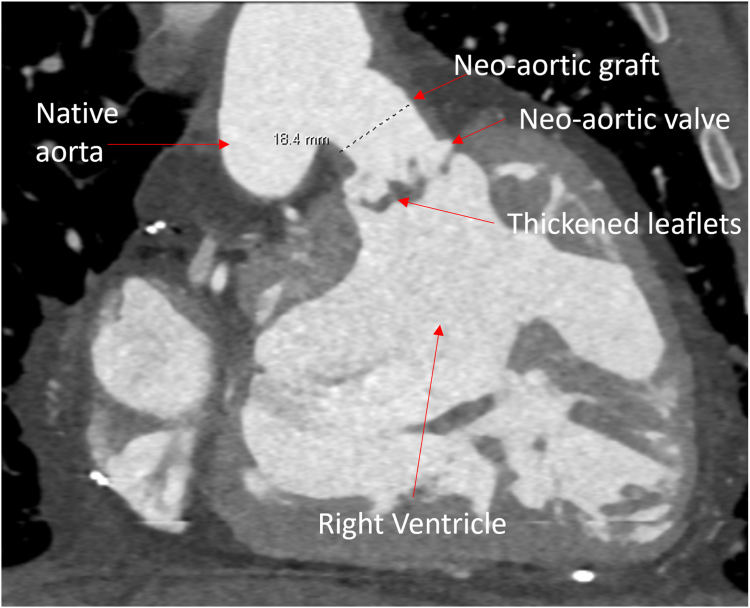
Frontal projection from cardiac computed tomography showing the native aorta and its connection with the neoaortic hemashield graft. The graft measures 18 mm in diameter. The neoaortic valve is seen with thickened leaflets.
